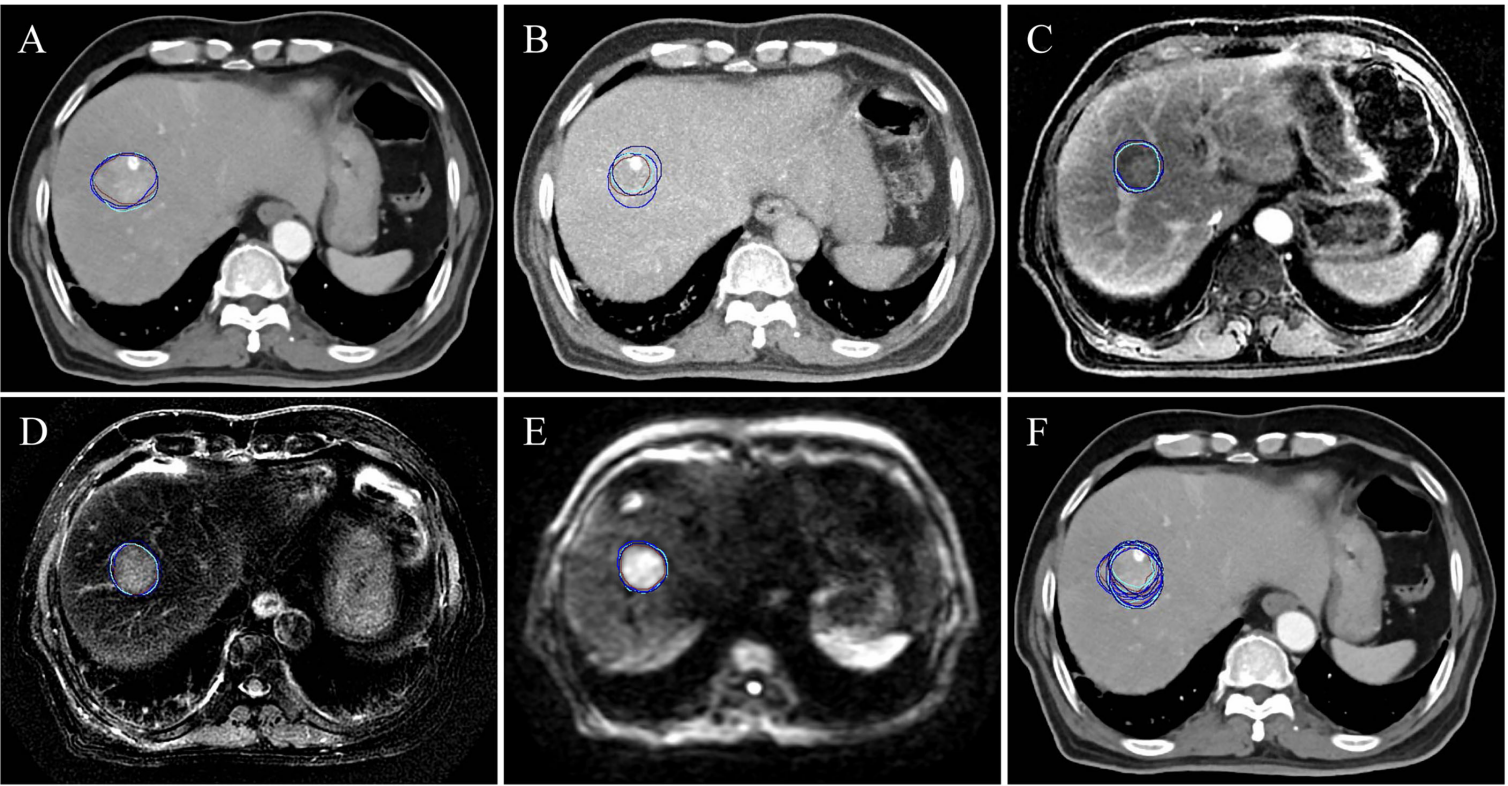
A subject (a male aged 69 and diagnosed with hepatocellular carcinoma) of the target delineation for primary liver cancer in 3D-CT, MIP and multiple-parameter MR images. (A-E) show the delineation results of CECT, MIP, LAVA-Flex, T2W FRFSE and DWI-EPI, respectively. (F) shows all the delineation results displayed on CECT. The blue, brown, cyan and dark blue contour denote the first delineation of the first observer. The brown contour is the second delineation of the first observer. The cyan contour is the first delineation of the second observer. The dark blue is the second delineation of the second observer.
